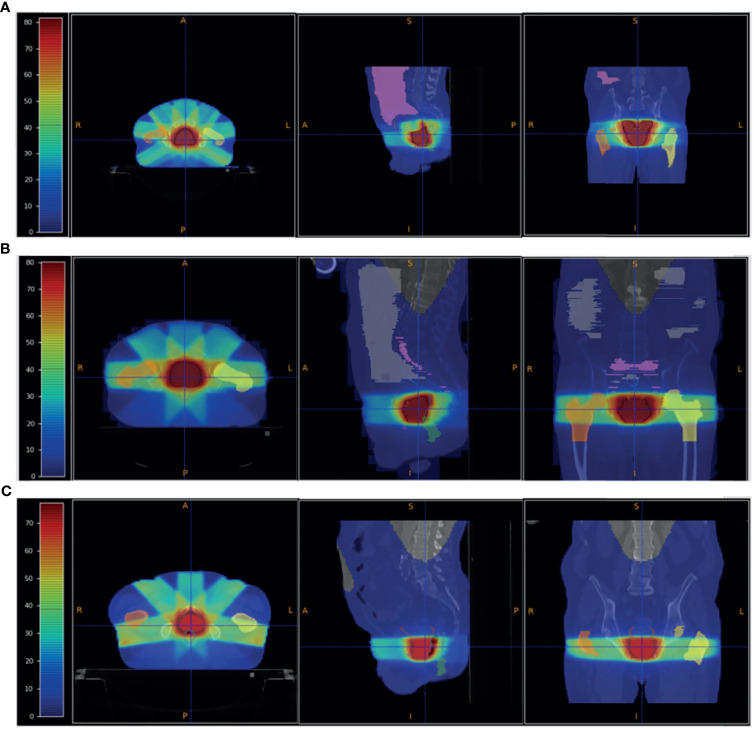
Patient PZ1 (A), PZ2 (B) and PZ3 (C) CT overlapped with the dose map optimised using the Pinnacle TPS software for an IMRT treatment using respectively 7 (PZ1) and 5 (PZ2 and PZ3) photon beams. The OARs are shown: the femurs in yellow and orange, the bladder surface in brown, the rectum surface in dark blue. The PTV is shown in red. The absorbed dose related to the full treatment (39 fractions for PZ1 and PZ2, and 38 fractions for PZ3 of 2 Gy each) is shown.
